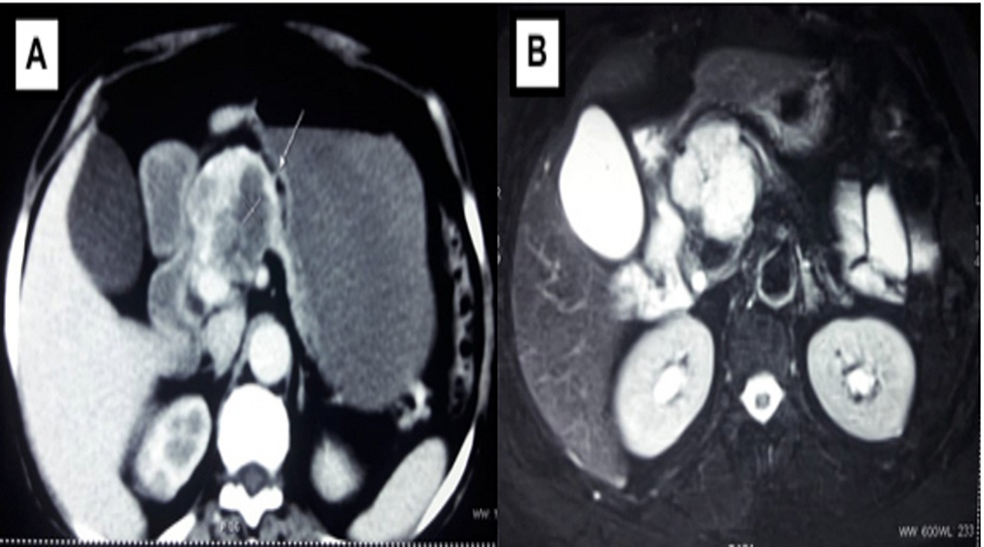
A & B: CT and MRI picture showing a heterogeneously enhancing cystic lesion in the neck of the pancreas
